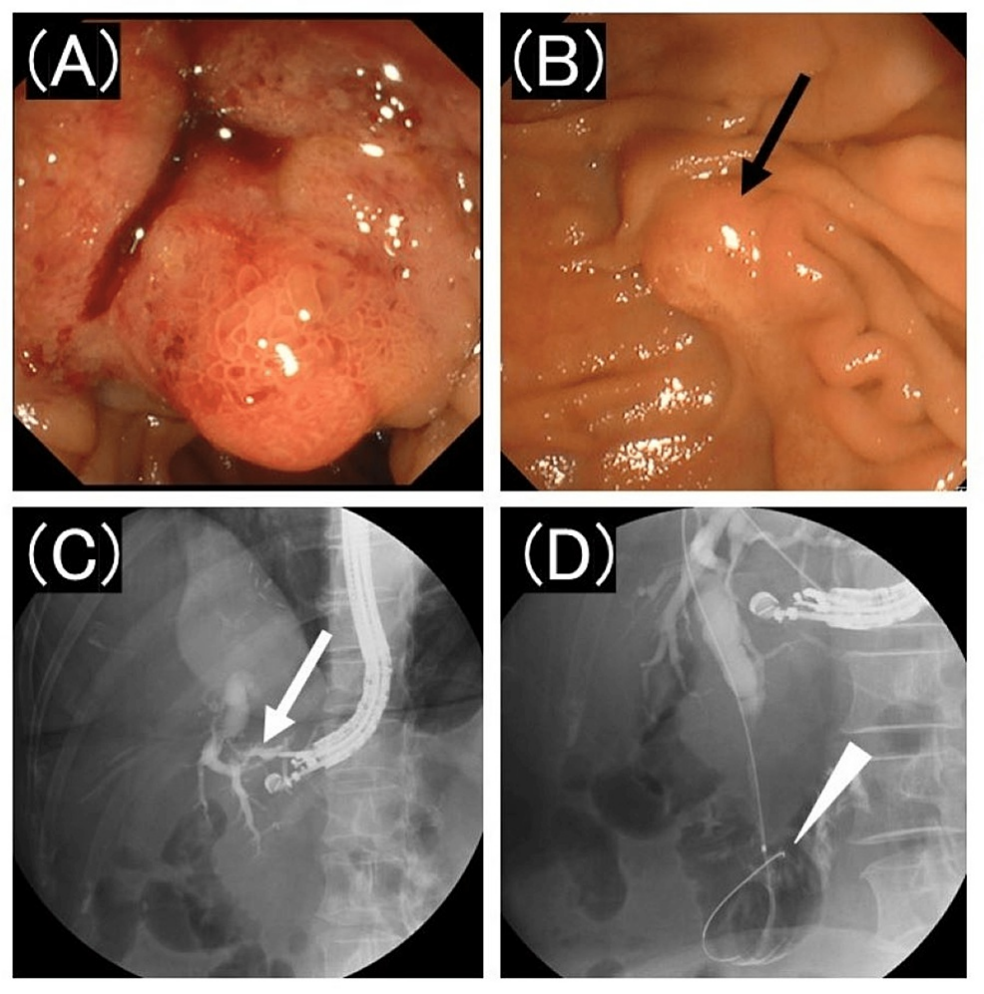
Image of the duodenum during endoscope insertion and fluoroscopic image during endoscopic ultrasound-guided hepaticogastrostomy (A) The pancreatic tumor invades the duodenum. (B) The endoscope successfully reaches the duodenal papillae (black arrow). Then, attempts to insert a catheter into the bile duct prove unsuccessful. (C) An echoendoscope is inserted, and the B3 segment of the intrahepatic bile duct (white arrow) is punctured transgastrically with a 19-gauge needle. The guidewire is then inserted into the bile duct. (D) The guidewire (arrowhead) is easily guided through the duodenal papillae and into the duodenal lumen.
